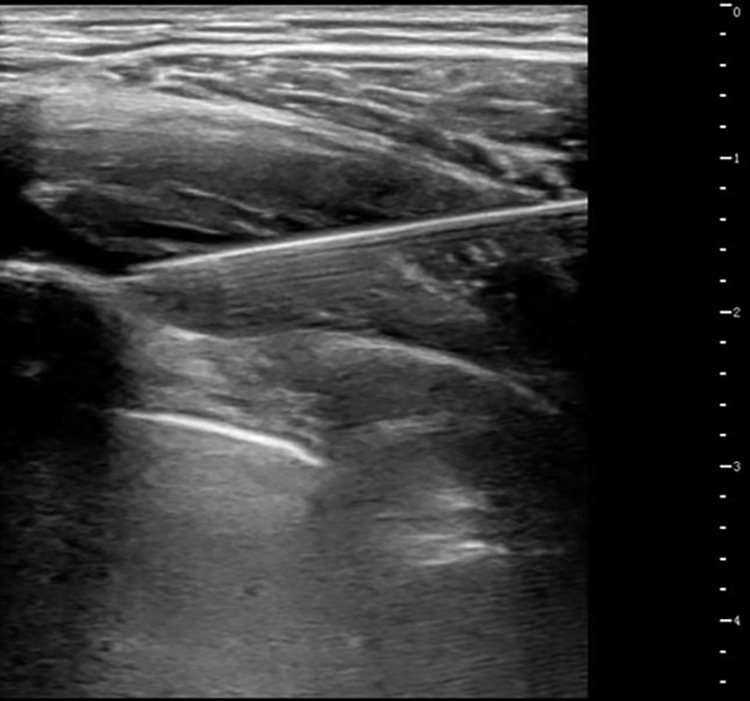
Ultrasound-guided SAPB. After injection, a hypoechoic fluid area is visible within the fascial plane between the inferior surface of the serratus anterior muscle and the superior surface formed by the ribs and external intercostal muscles.
